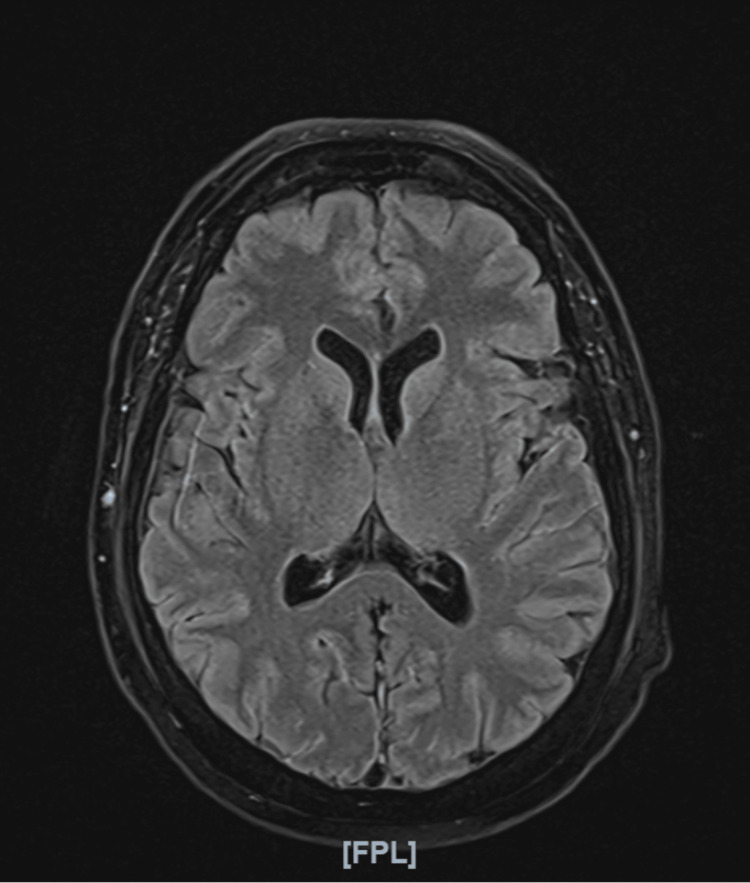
Axial T2 FLAIR MRI of the brain showing no acute intracranial abnormalities FLAIR: Fluid-attenuated inversion recovery
